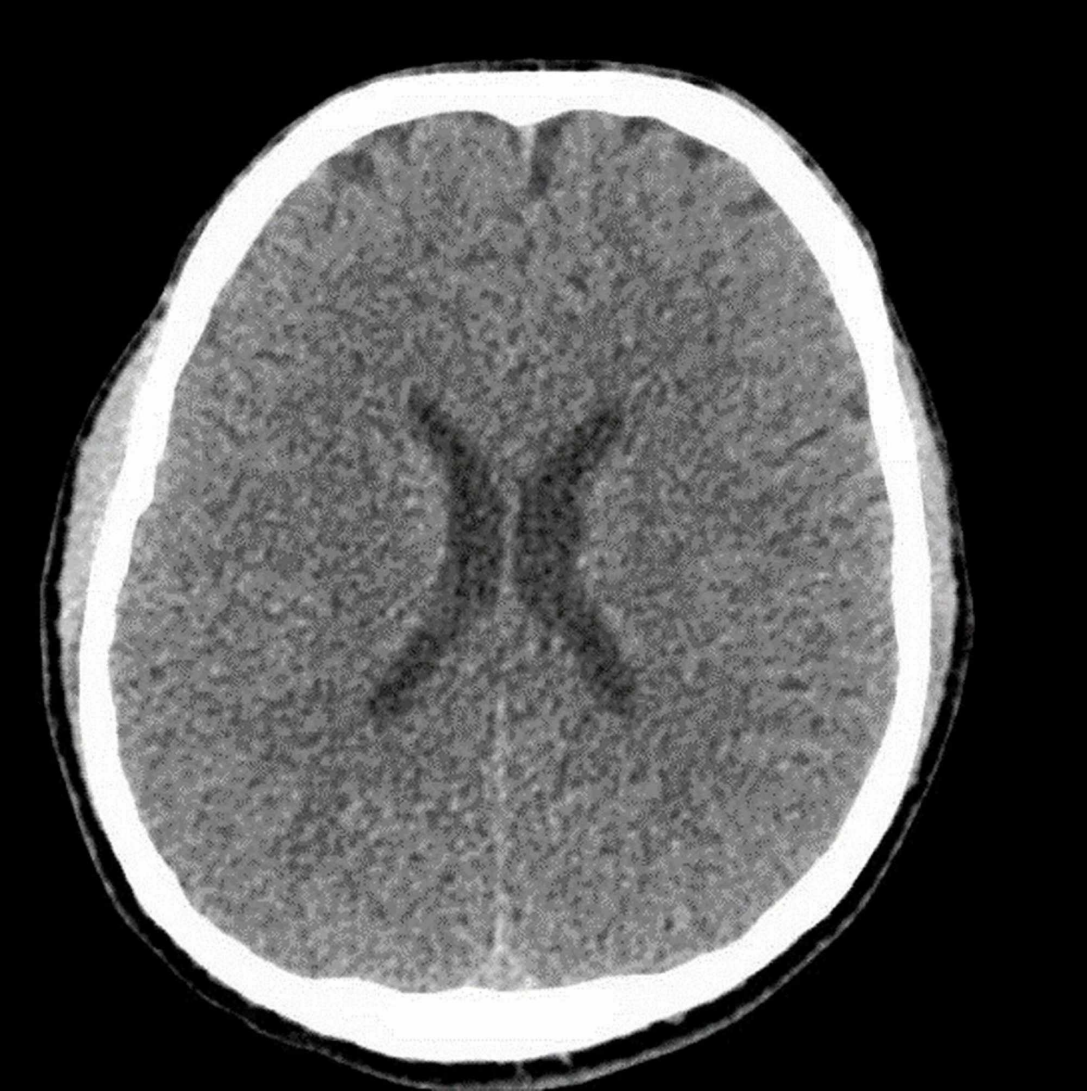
CT scan of the brain: unremarkable for any acute insult.
